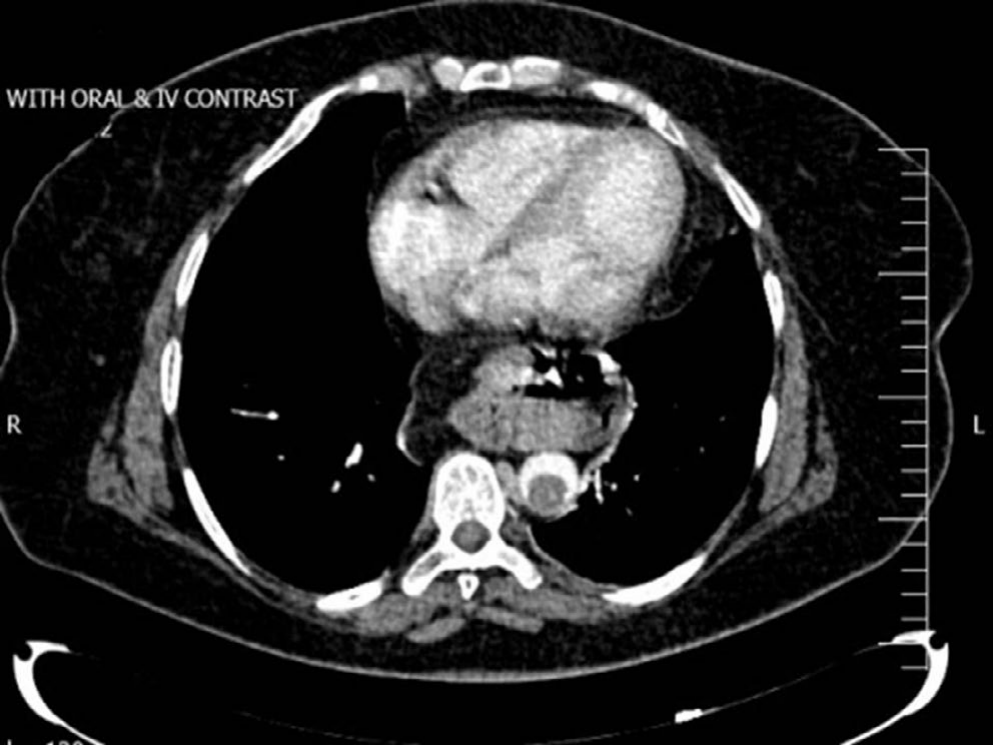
Abdominopelvic CT scan with oral and IV contrast, axial view: a floating thrombus in aortic artery lumen with transverse diameter of 16 mm
